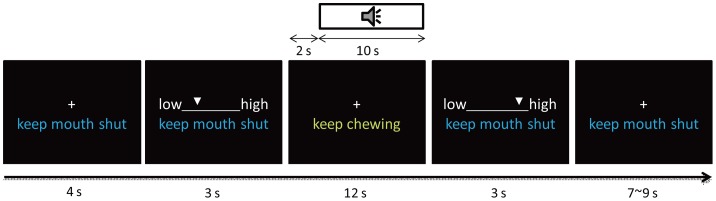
Sequence of events in a trial for functional MRI scanning.
